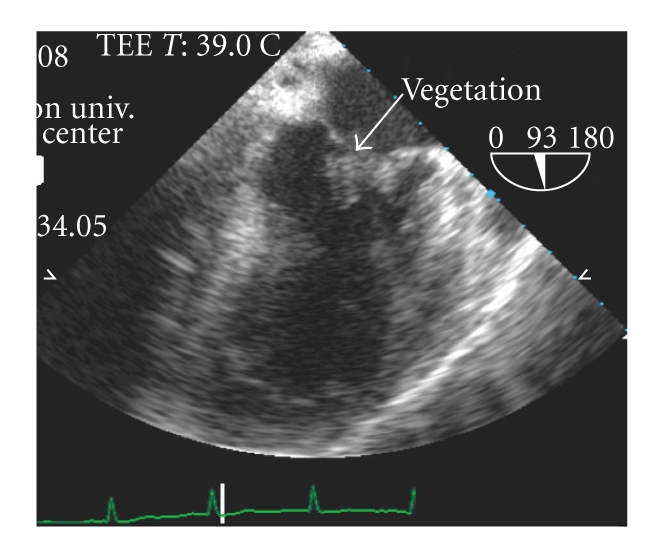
Transesophageal echocardiogram revealing vegetation on the mitral valve leaflets.
